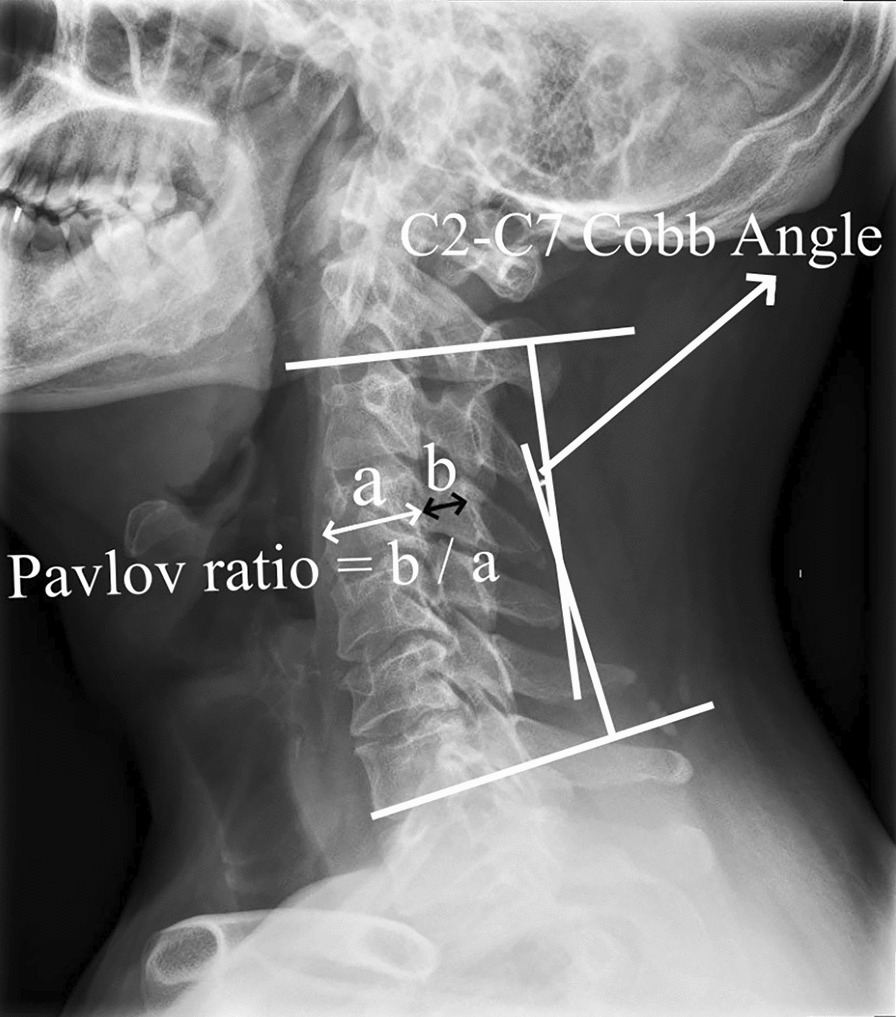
Preoperative radiographs of mCSM with DCS; the figure shows the measures of the Pavlov ratio and the C2–C7 Cobb angle (angle of intersection of C2 and the extension of the lower end plate of C7)
